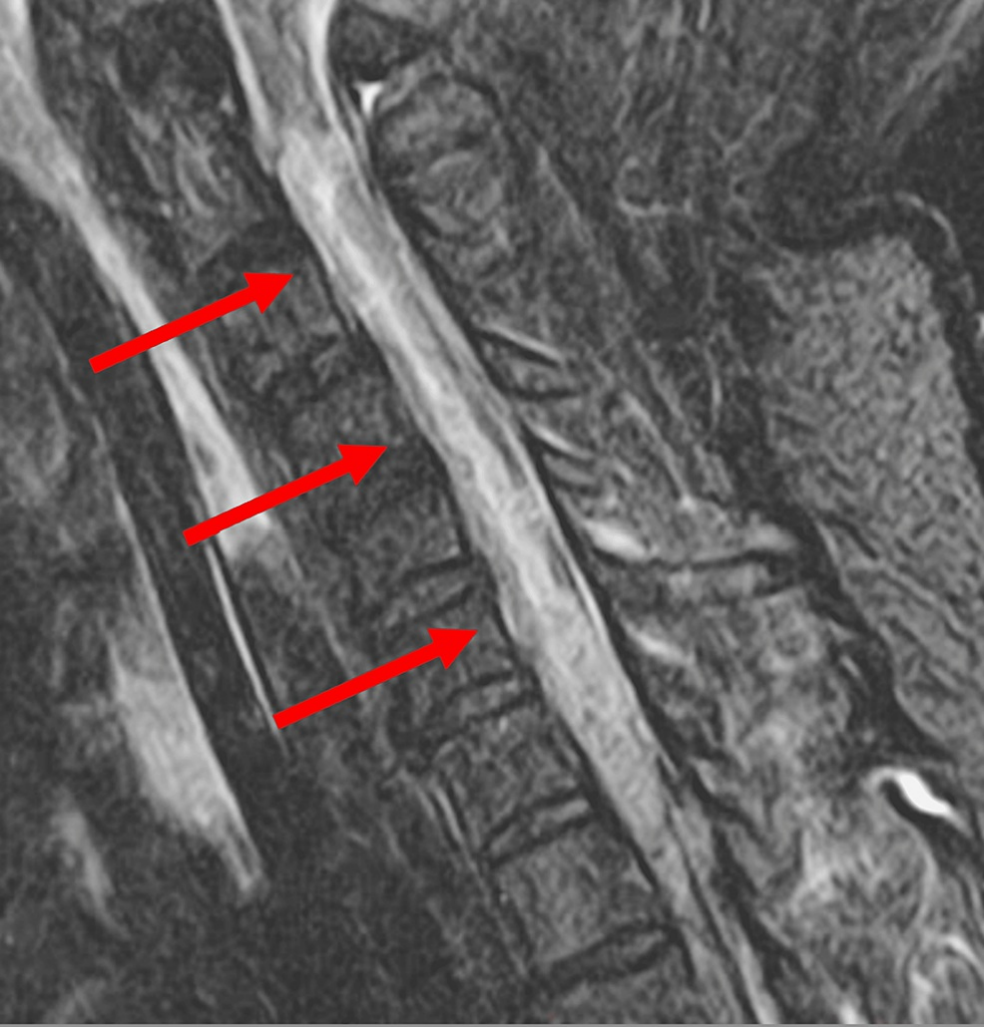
Transverse Myelitis visualized on MRI Red arrows point to abnormal T2 signaling and increased cord expansion extending from C2-C7.
